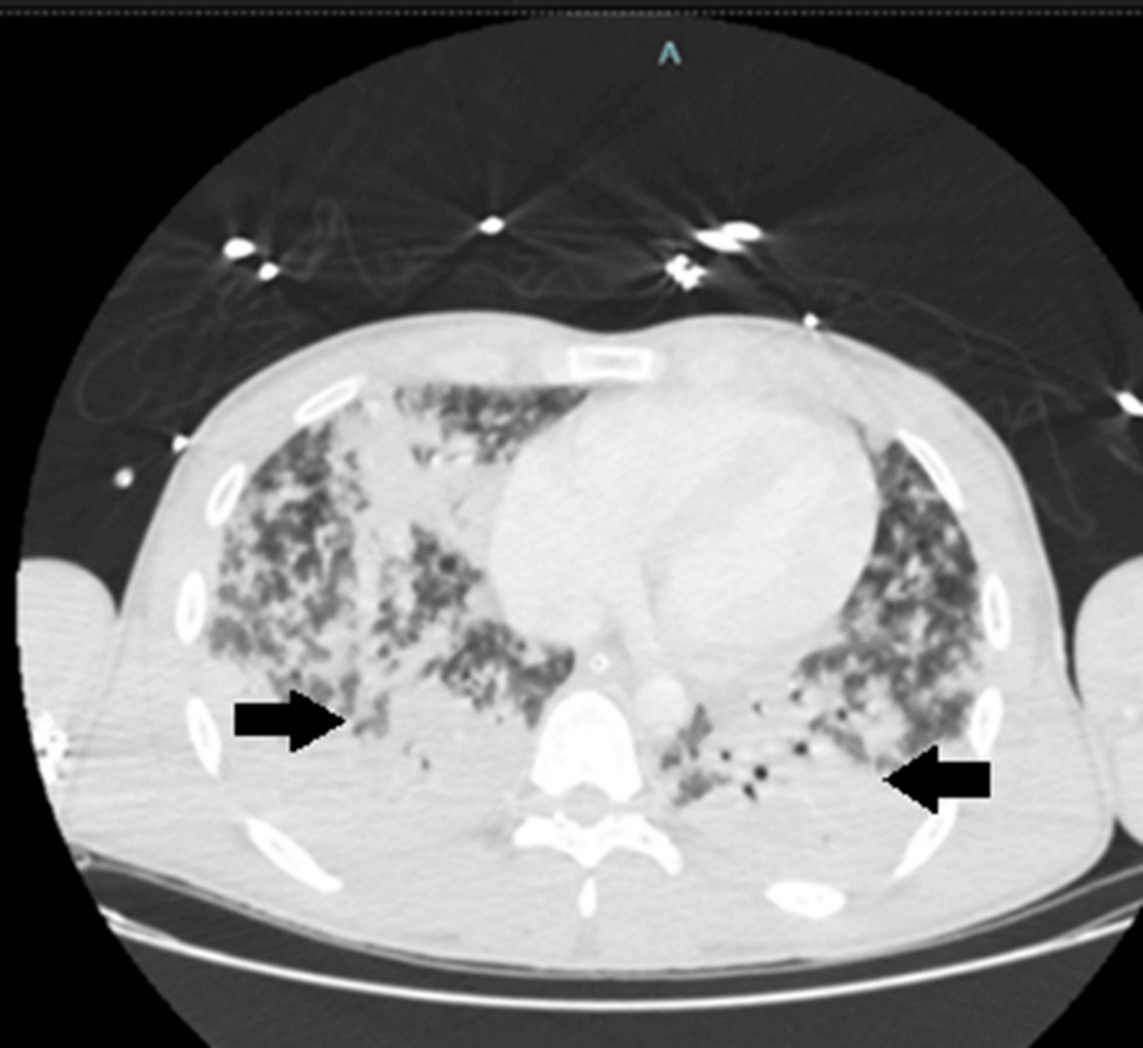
Thoracic CT showing bilateral lung consolidations
